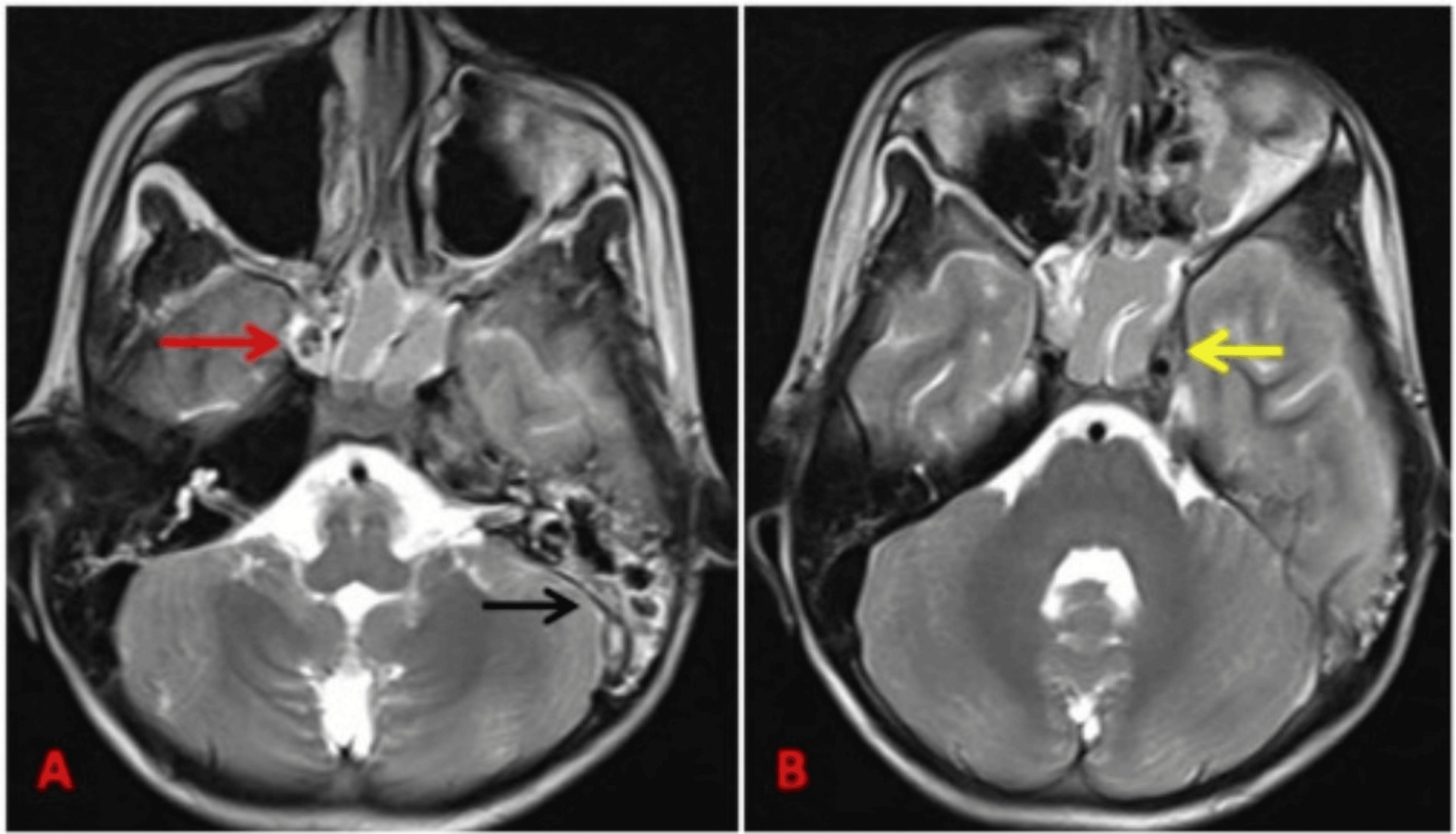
(A) Axial T2 showing sphenoid sinusitis appearing hyperintense on T2-weighted images with inspissated secretions appearing hypointense (marked by red arrow). Left mastoiditis showing hyperintense fluid collection on T2-weighted images (marked by black arrow). (B) Axial T2 showing bulky left cavernous sinus with outer convex margin (marked by yellow arrow).
